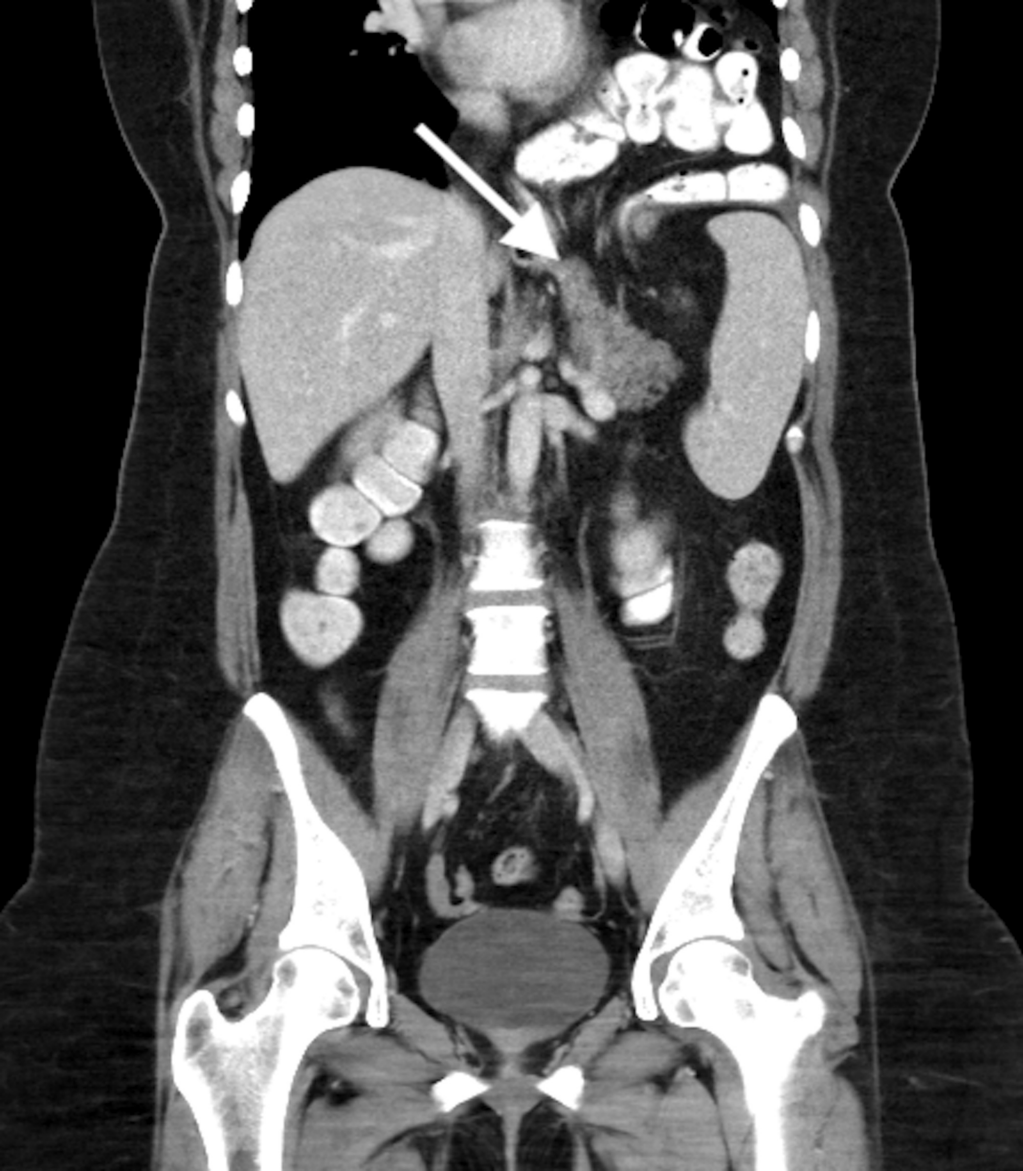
CT-scan of Patient C depicting herniation of the pancreas into the thoracic cavity.
